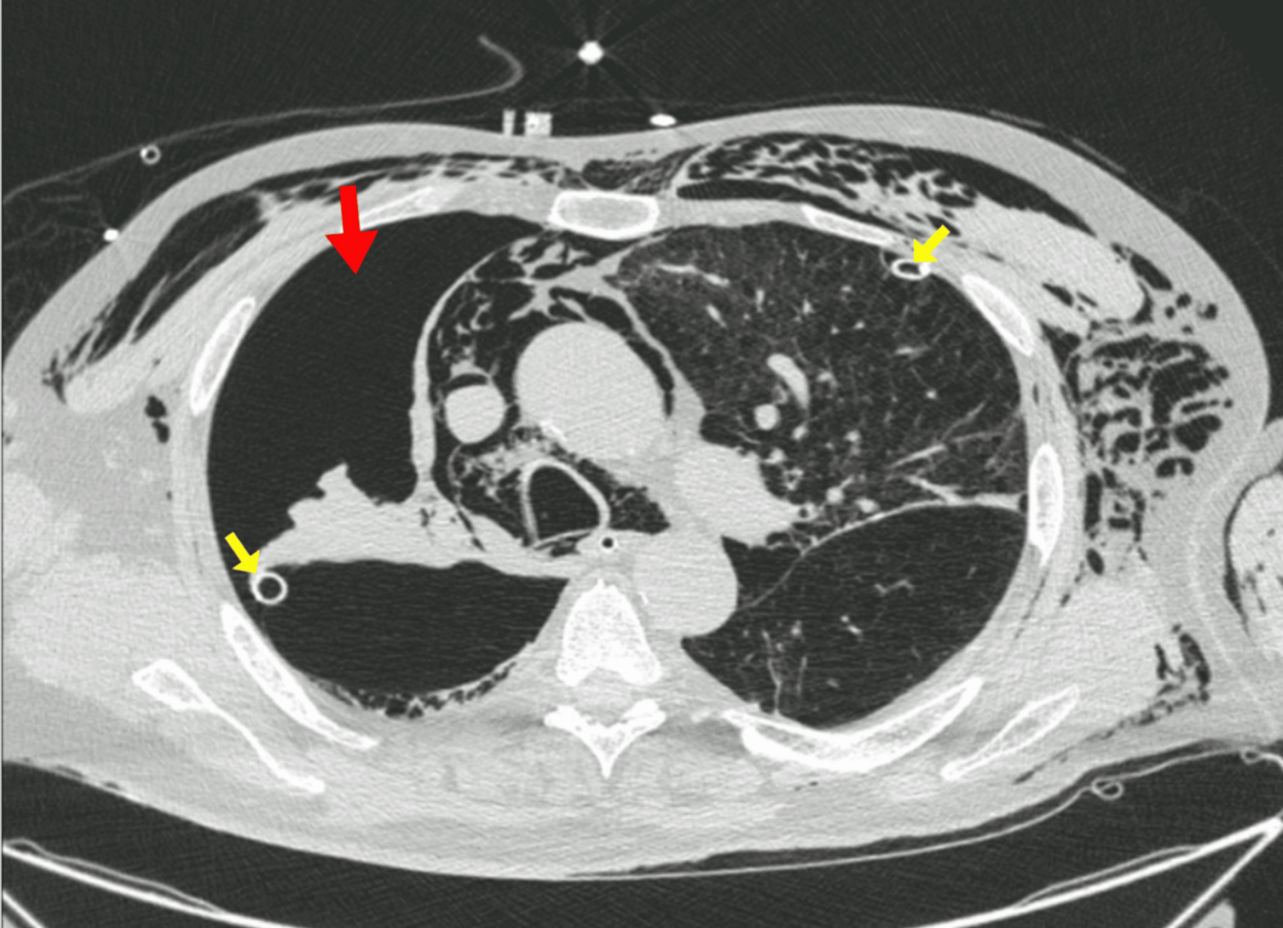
Axial chest computed tomography showing persistent right-sided tension pneumothorax after chest tube placement Axial chest computed tomography showing a persistent large right-sided pneumothorax (red arrow) with incomplete lung re-expansion despite chest tube drainage (yellow arrows), corresponding to the lung treated with endobronchial valve placement.
